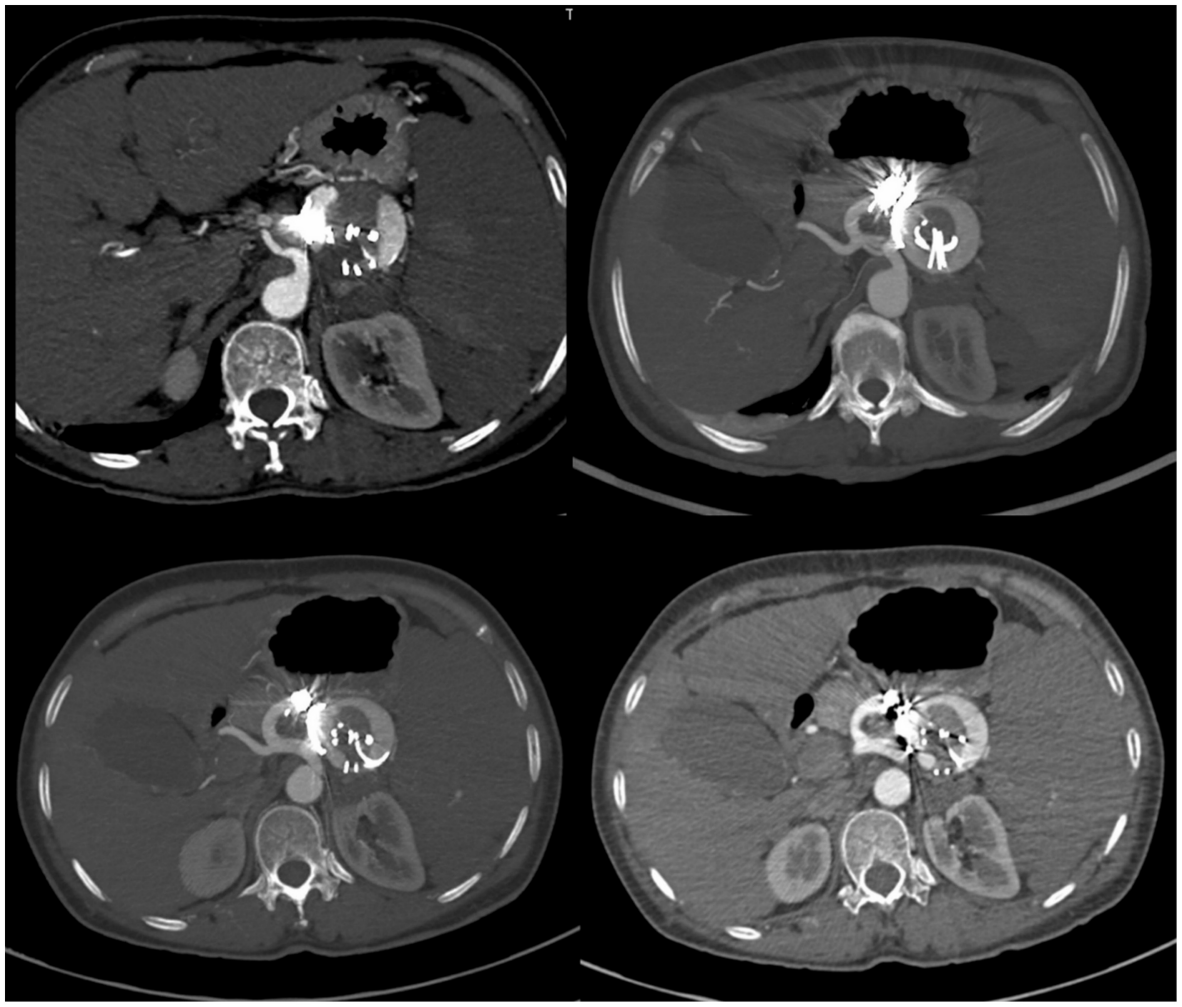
CT-scan of the abdomen showed signs of blood flow into the embolized splenic aneurysm sac.
